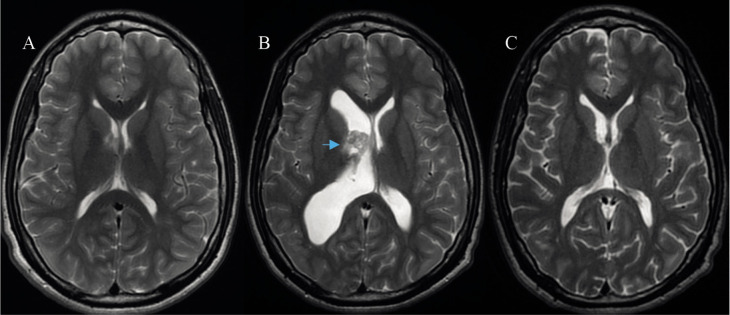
Brain MRI study, axial T2 cuts. Image A: Initial study showing normal ventricular size. Image B: Four years later compared to Image A, showing the presence of right monoventricular hydrocephalus and SEGA (blue arrow). Image C: Postoperative study displaying complete resection of the lesion and normalization of ventricular size.
